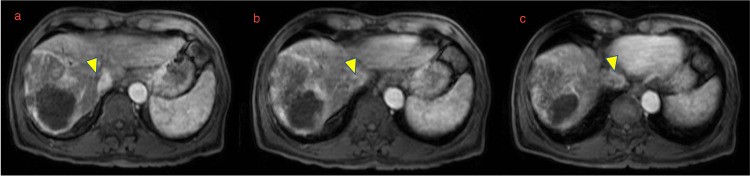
Sequential axial contrast-enhanced magnetic resonance imaging demonstrating tumor thrombus progression Sequential axial contrast-enhanced magnetic resonance imaging demonstrating (a) tumor thrombus within the right hepatic vein, (b) cranial progression of the thrombus toward the hepatocaval confluence, and (c) extension of the thrombus into the inferior vena cava. Arrowheads indicate the tumor thrombus.
